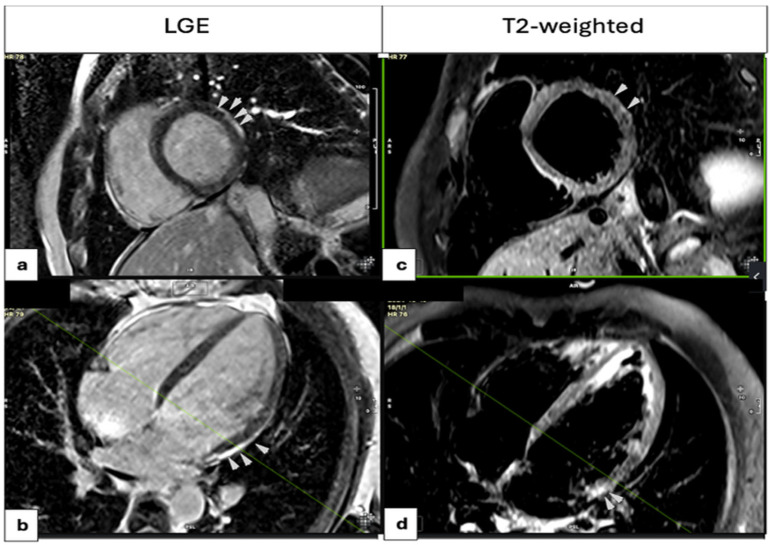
LGE distribution (a,b) and T2-weighted images (c,d). High-intensity signal (white arrows in the basal anterolateral wall segment on T2-weighted images (c,d)) indicates myocardial edema, with corresponding LGE in the same regions (a,b), findings consistent with acute myocarditis.
